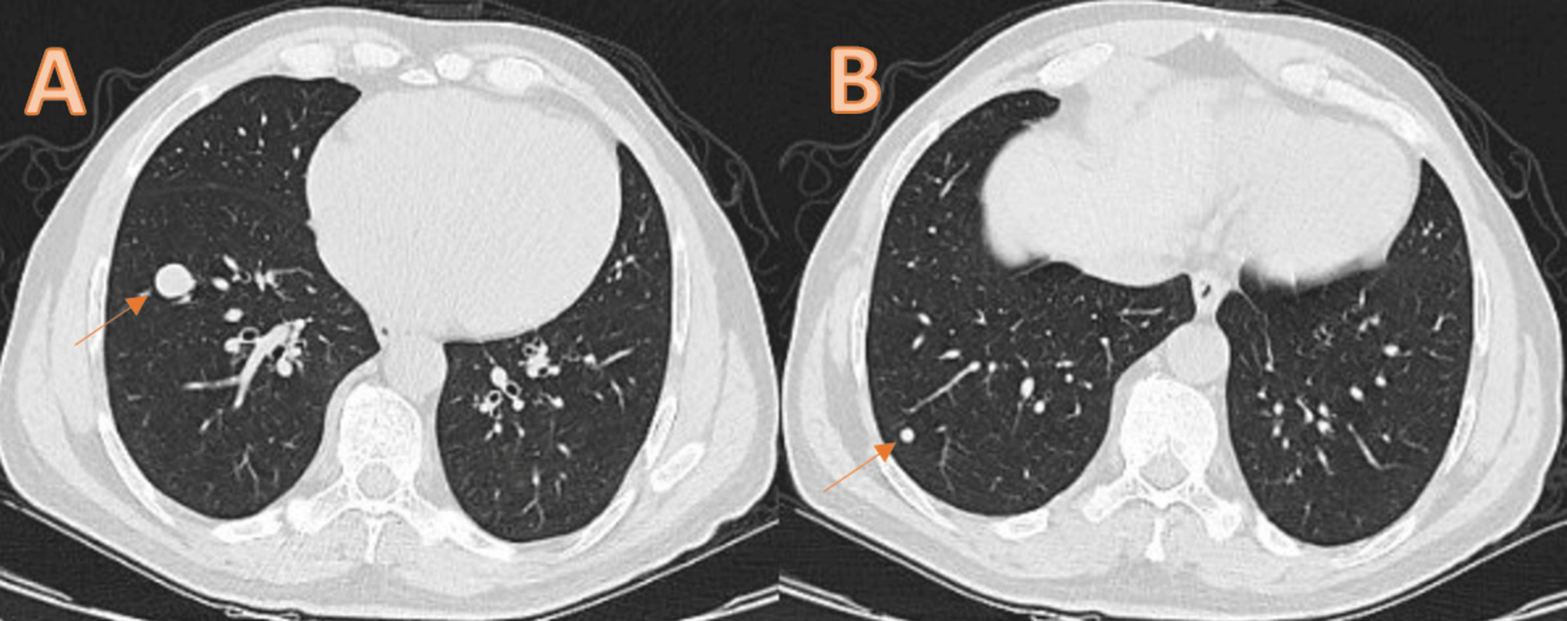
Pulmonary nodules detected on chest CT. (A) The arrow indicates a 14 × 12 mm nodule in the lateral basal segment of the right lower lobe. (B) The arrow indicates a 5 mm nodule in the posterior basal segment of the right lower lobe. CT, computed tomography
